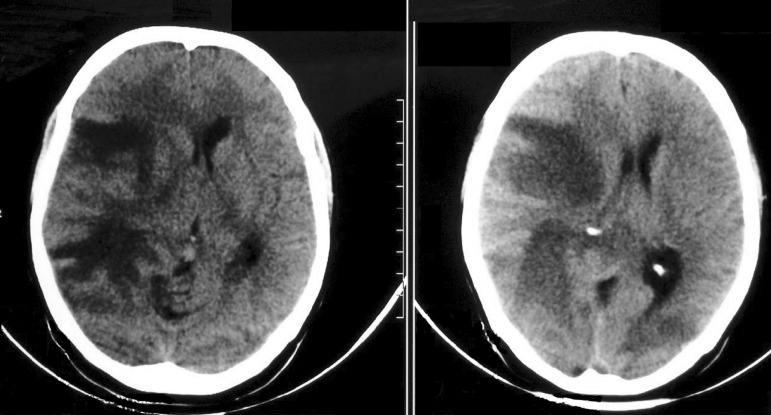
Head computed tomography showing a hypodensity area in the right
